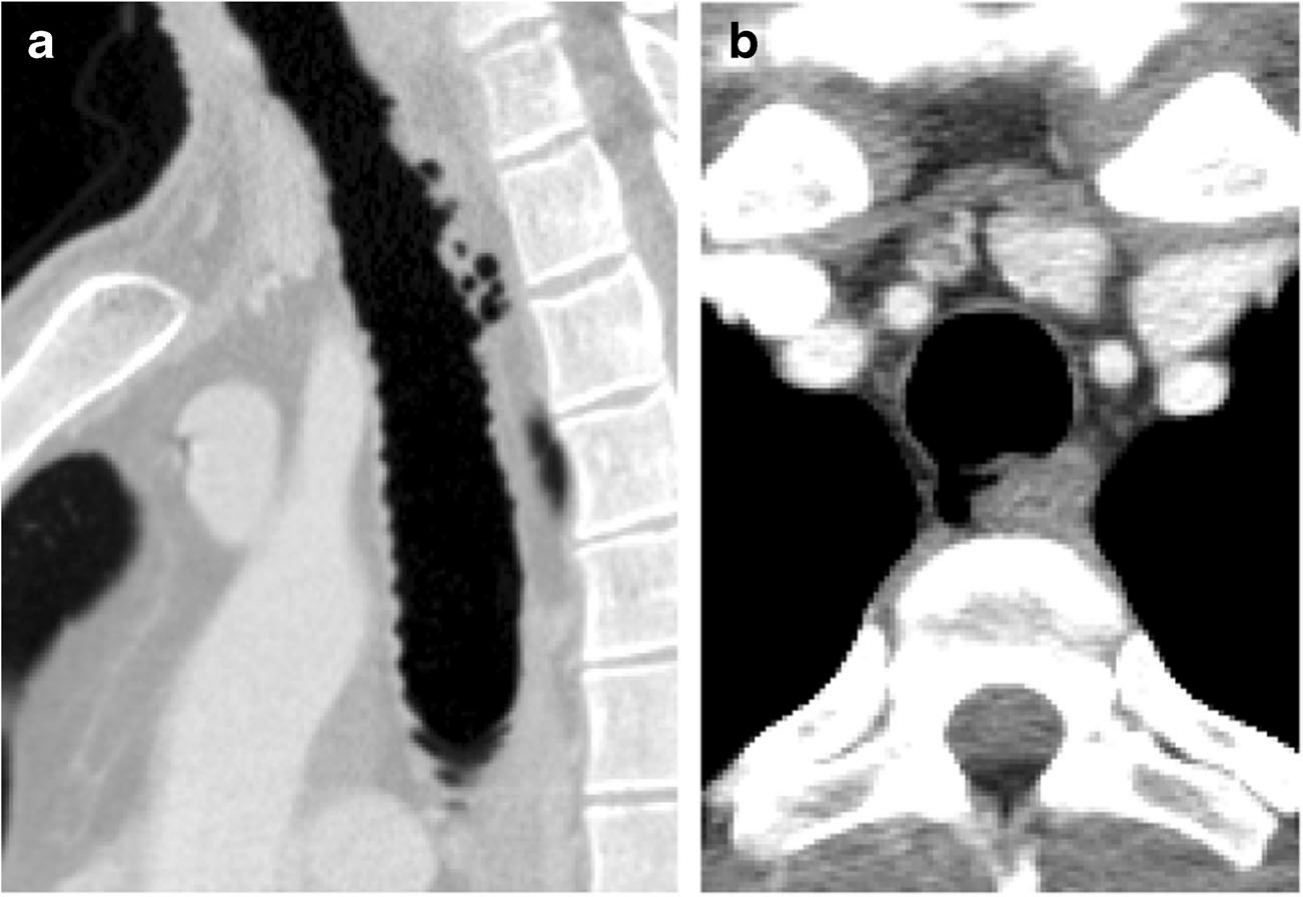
Thirty-nine-year-old man with a sagittal and b axial CT imaging demonstrating innumerable small diverticuli and corrugated appearance of the trachea compatible with tracheal diverticulosis
